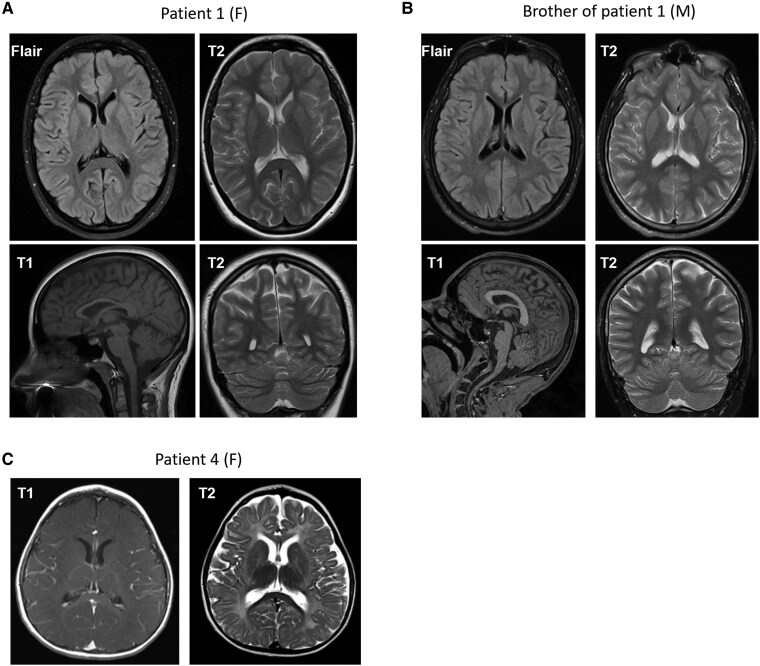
Magnetic resonance imaging (MRI) studies in patient P1 (female), her affected brother (patient P1B) and patient P4 (female). A, FLAIR-, T1-, and T2-weighted MRI of the brain of patient P1 performed at age 21 years, showing mild cortical atrophy without signs of leukodystrophy. B, FLAIR-, T1-, and T2-weighted MRI of the brain of the brother of patient P1 (patient IB) performed at age 25 years, showing signs of mild cortical atrophy without obvious signs of leukodystrophy. C, T1- and T2-weighted MRI of the brain of patient P4 at age 1 year show a small anterior cranial fossa and 6 to 7 month delayed myelination with nonmyelination in the superior and middle frontal gyrus. The applied MRI sequences are indicated in each image.
